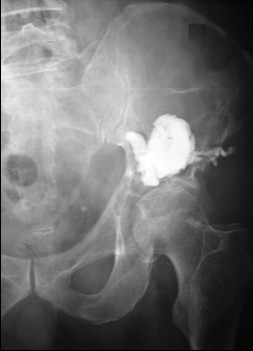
Postoperative plain radiographs.
